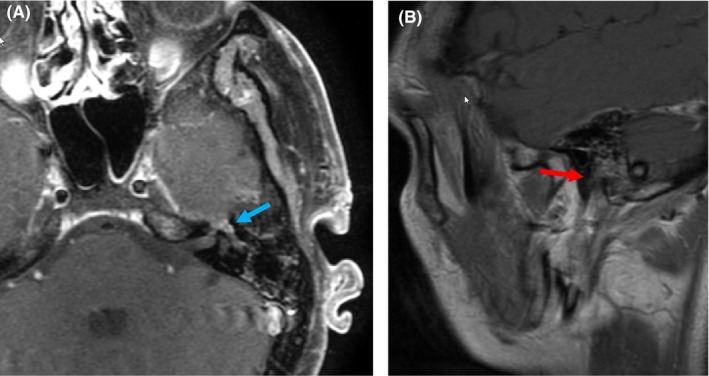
Follow‐up imaging status post completion of multimodality therapy. A, Axial and B, sagittal postcontrast, fat‐suppressed T1‐weighted MR images 12 months after completion of therapy, showing some mildly persistent but clearly diminished tumor enhancement in the left geniculate ganglion (blue arrow), and significantly regressed enhancement of the tympanic segment (compare with Figure 1C). There is near complete resolution of enhancing perineural tumor along the descending or mastoid segment (red arrow, compare with Figure 2B)
